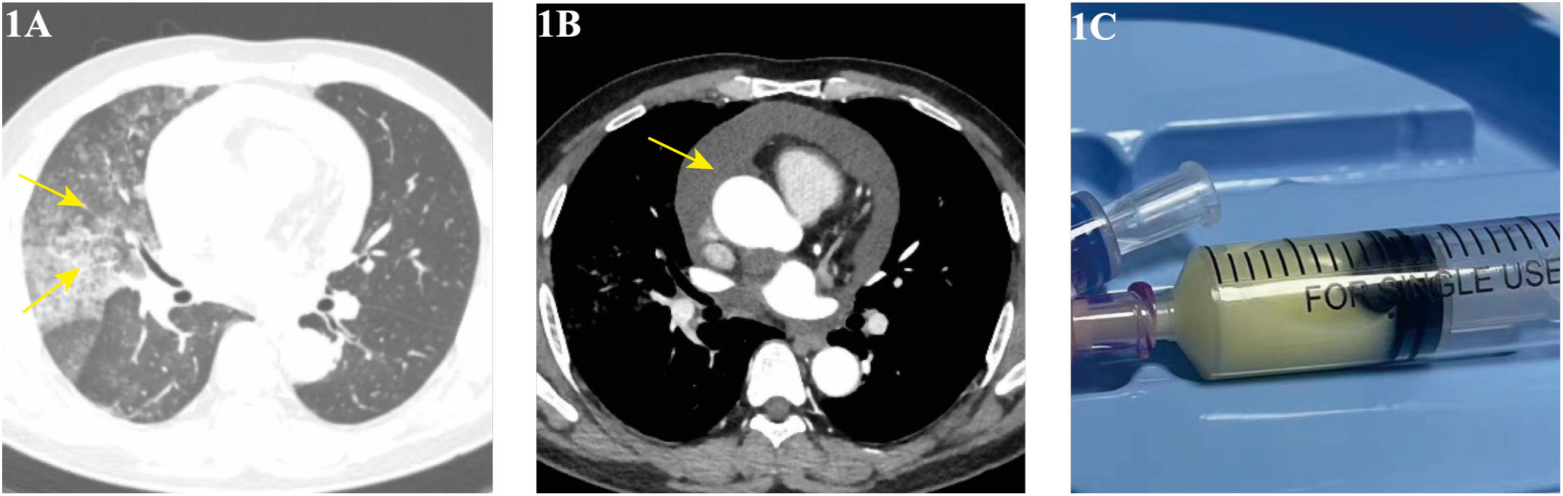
(A,B) Chest CT (August 29, 2023) showing right lung infiltrates and pericardial effusion; (C) pericardiocentesis yielding chylous fluid.
